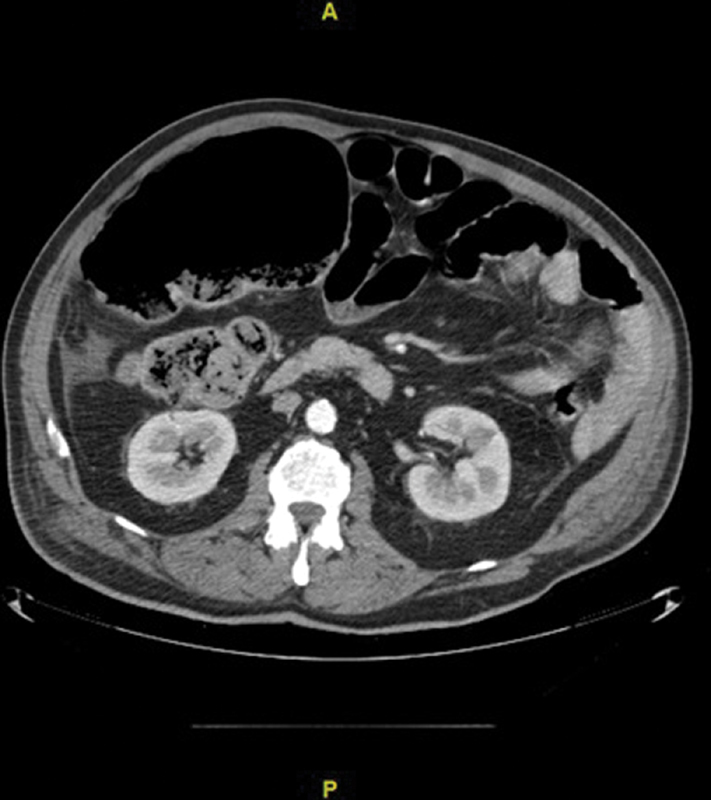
Abnormally distended cecum on axial view of CT abdomen/pelvis.
